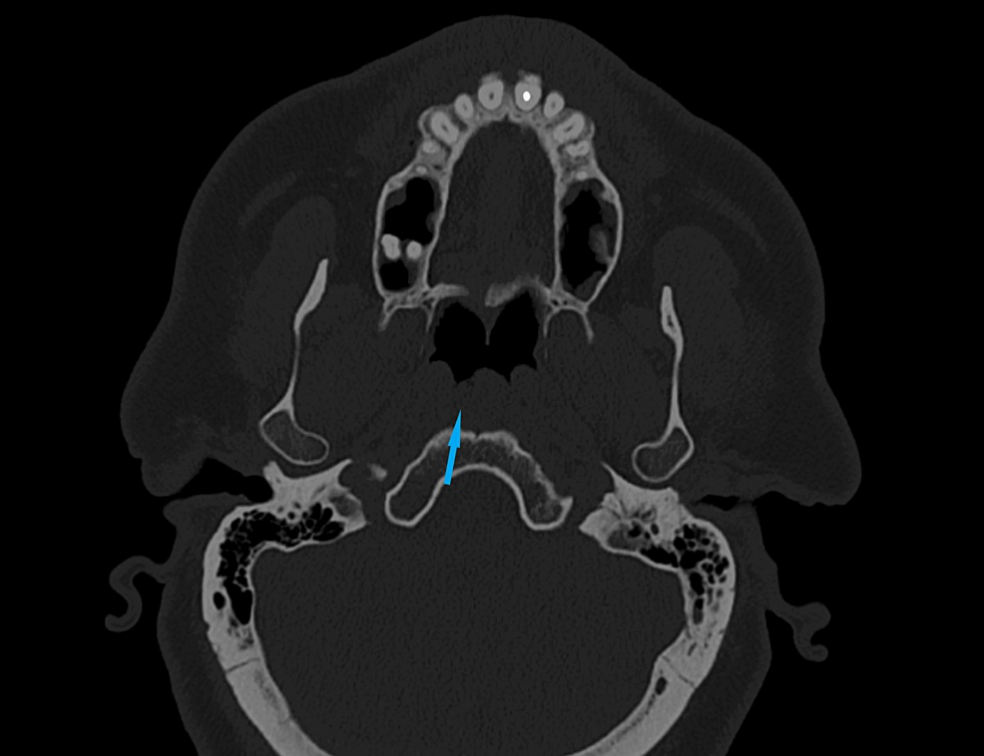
Computed tomography six months post-endoscopic marsupialization of Tornwaldt cyst. Blue arrow showing the absence of a lesion in the nasopharynx.
